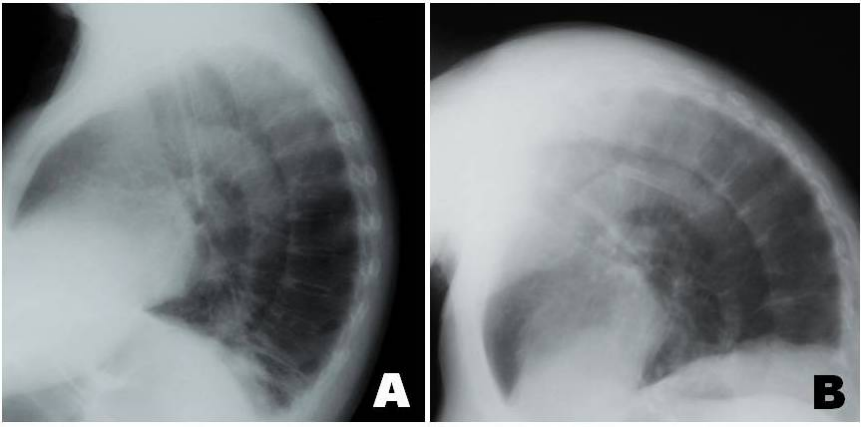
Chest X-rays in supine position at one-week interval showing (A) porotic kyphosis and (B) camptocomic posture.
